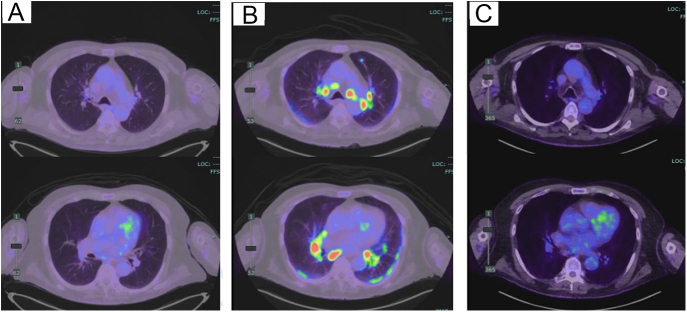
Axial CT/PET (A) before immunotherapy, (B) after immunotherapy and (C) after prednisolone.
